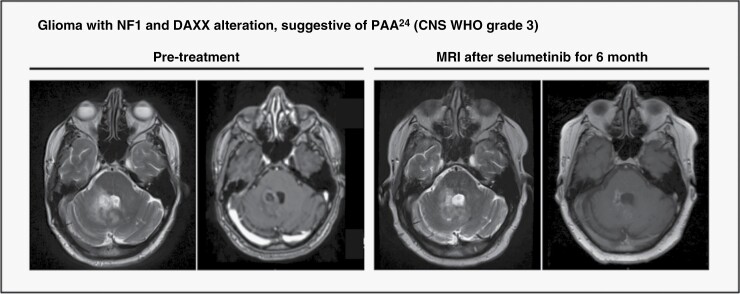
Case example of a male patient with a Glioma with NF-1 and DAXX alteration, suggestive of PAA.^24^ Baseline MRI scan (T2 weighted and contrast-enhanced T1 weighted sequences) at progression after CCNU alkylating chemotherapy and 6-month follow-up MRI scan (T2 weighted and contrast-enhanced T1 weighted sequences) following MEKi Selumetinib revealing partial response.
